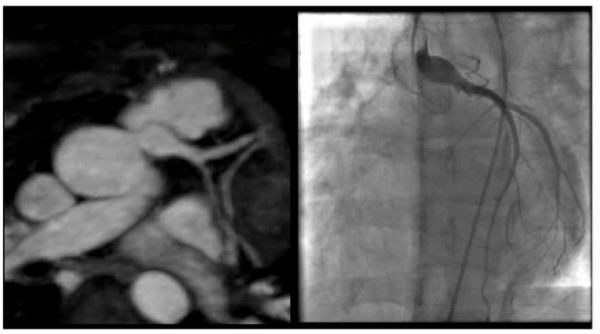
3D b-SSFP whole heart images, magnetic resonance coronary angiography MRCA (left) shows large patent, reconstructed, re-implanted left coronary artery into the left coronary sinus. Findings confirmed on X-ray coronary angiography (right).
